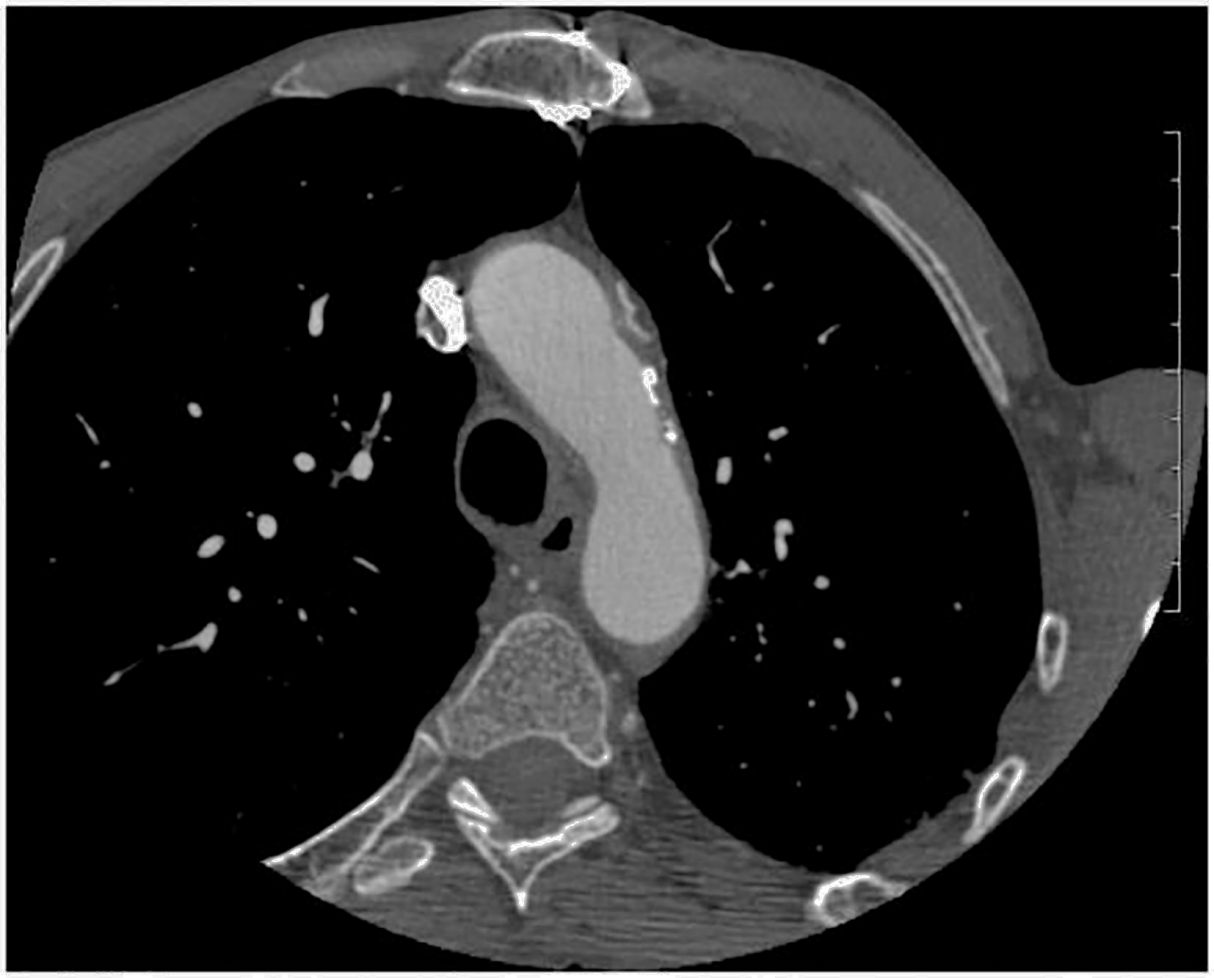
Computed tomography angiography showing an AAA.
